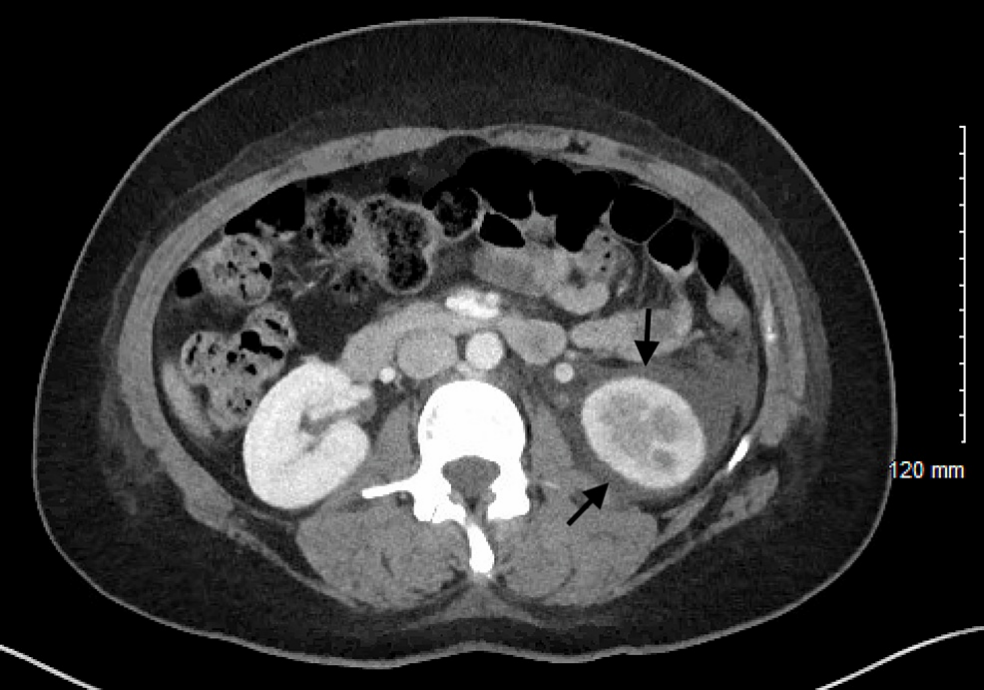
Computed tomography (CT) abdomen and pelvis with contrast showing abnormal hypo-enhancement of the inferior half of the left kidney with prominent perinephric fluid (arrows) in a patient with left renal vein thrombosis
